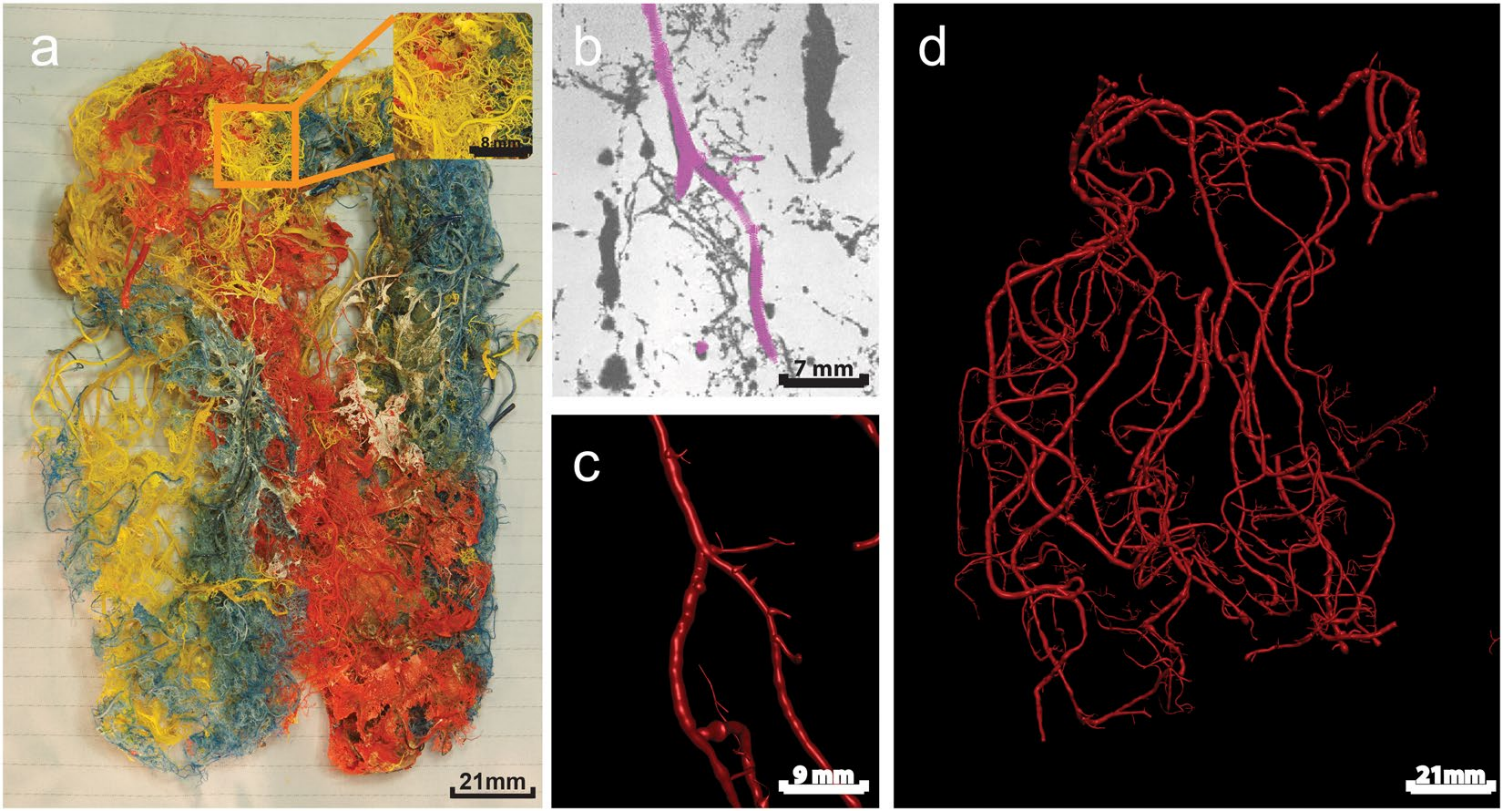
A human cerebral cast scanned with 7T MRI. (a) Photographic image of the complete cast. The inset shows the enlarged section indicated by the orange square. Different pigments where used for different major cerebral arteries during injection of Araldite F. (b) Detail of a 7T MRI image (100 µm isotropic resolution), with segmented artery as displayed in Simple Neurite Tracer. (c) The segmented artery of panel b in 3D representation. (d) 3D visualisation of all reconstructed arteries. As the reconstruction approach was semi-automatic not all arteries in the casts are segmented, only arteries which were manually selected in Simple Neurite Tracer are reconstructed to 3D.
